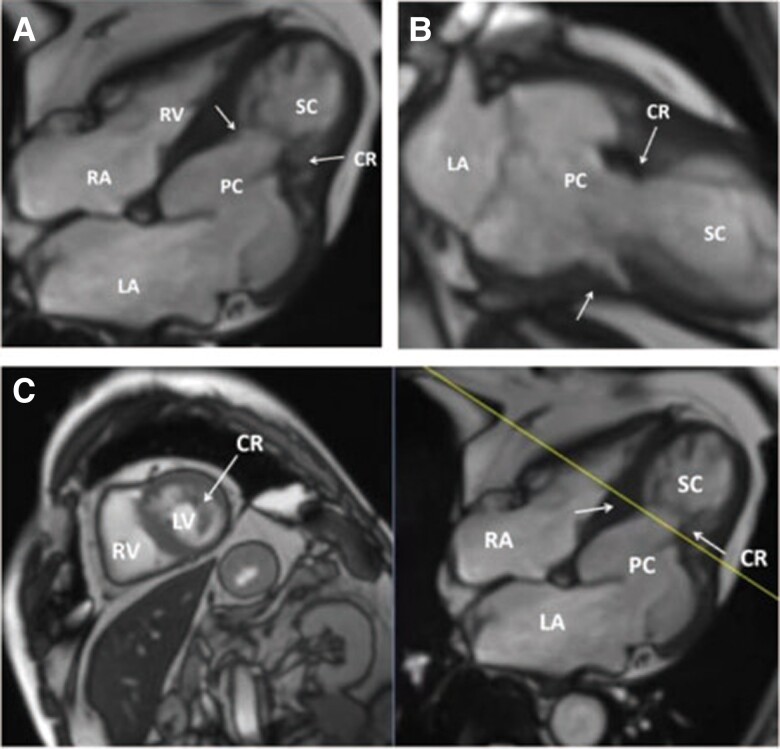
Cardiac magnetic resonance imaging. *(A)*, *(B)*, and *(C)*, Cardiac magnetic resonance showing apical four-chamber heart, apical two-chamber heart, and short-axis views corresponding to dividing muscular shelves.
